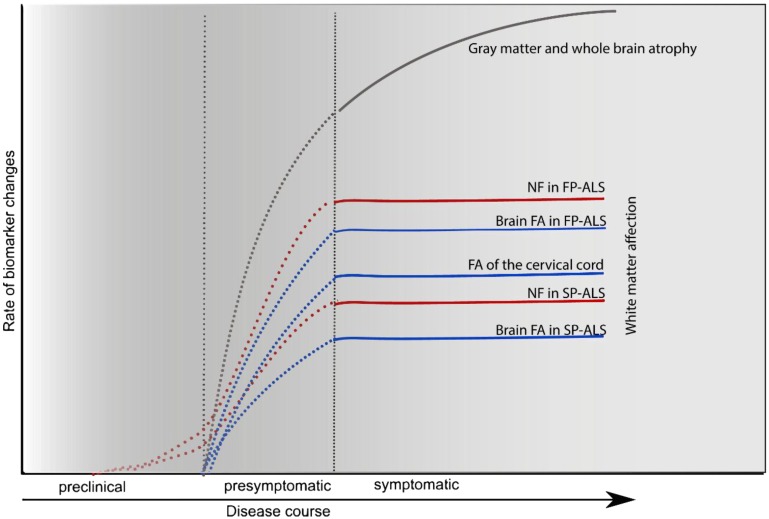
Dynamics of changes in the CSF and MRI-parameters of neurodegeneration over the course of the ALS. In different longitudinal imaging studies, the Fractional Anisotropy (FA) was relatively stable, but different between patients with fast (FP-ALS) and slow (SP-ALS) progressive ALS. The same studies showed progressive atrophy of the cortical grey matter and spinal cord in the symptomatic stages. This suggests that the major damage of corticospinal tract occurs in the presymptomatic stage of the disease. Accordingly, the rate of changes in FA and neurofilaments (NF) in the CSF would be greater in the presymptomatic stage rather than after appearance of the symptoms.
